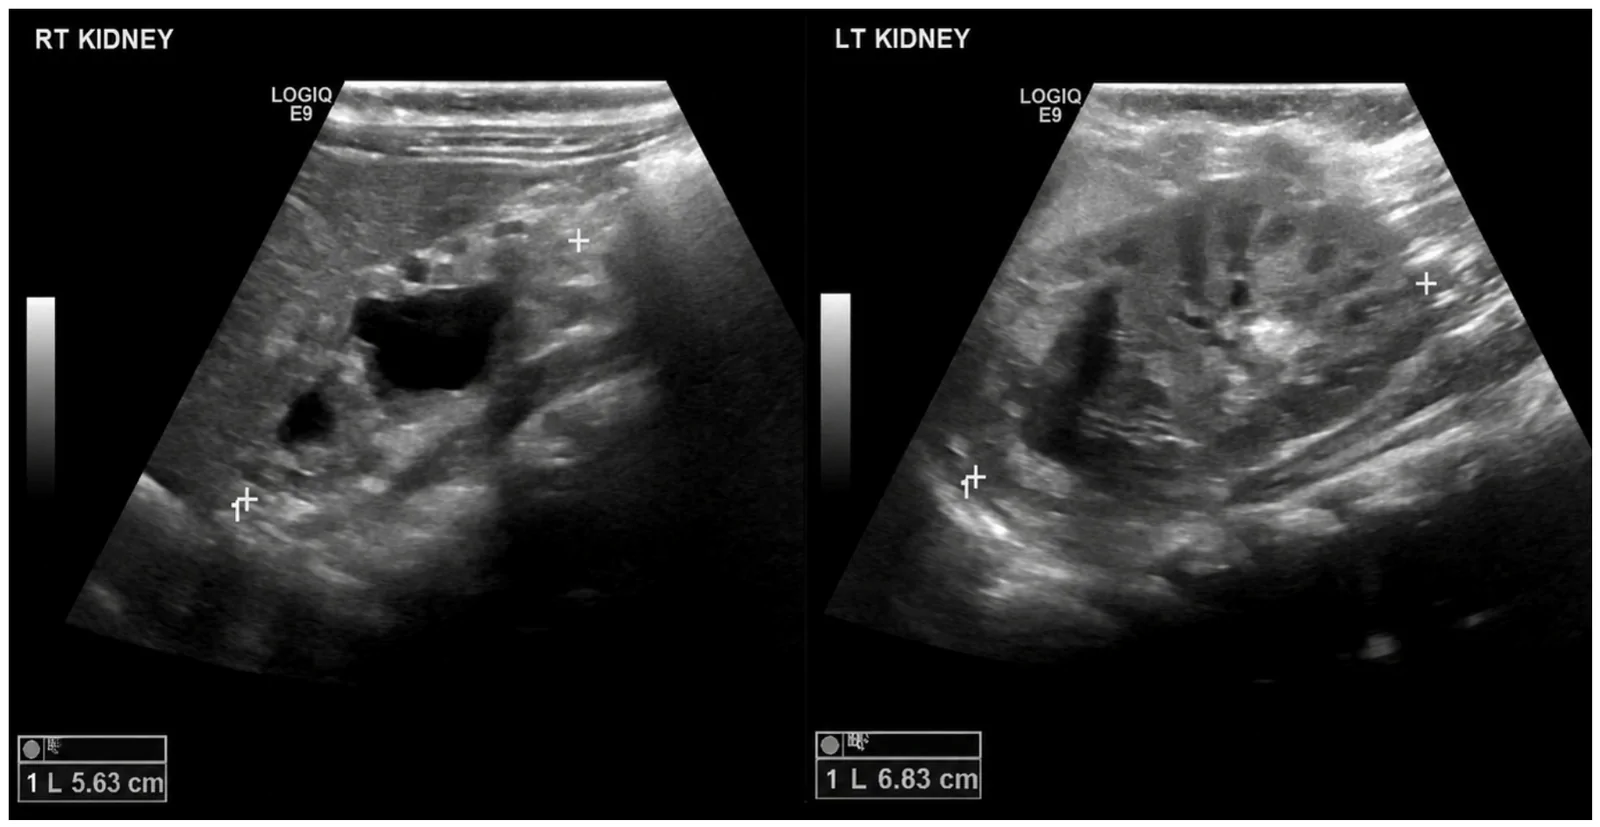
Follow-up renal ultrasound four months after nephrostomy removal demonstrating stable postoperative findings. Persistent size and multicystic dysplastic morphology of the right kidney.
